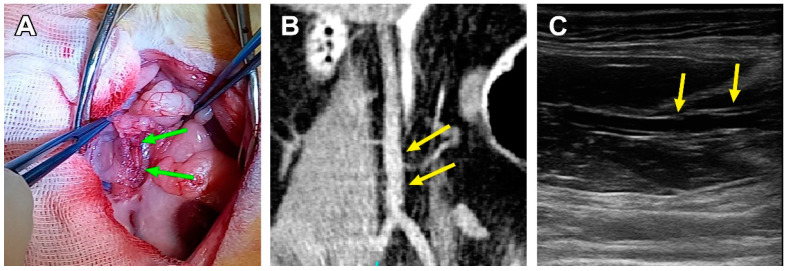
A patent PCL graft sample 180 days after implantation. (A) *Post-mortem* examination, green arrows indicate the margins of a PCL graft. (B) CBCT, coronal section, the yellow arrows indicate the margins of the graft. (C) An ultrasonic image of a patent scaffold, frontal section, the yellow arrows indicate the margins of the graft.
